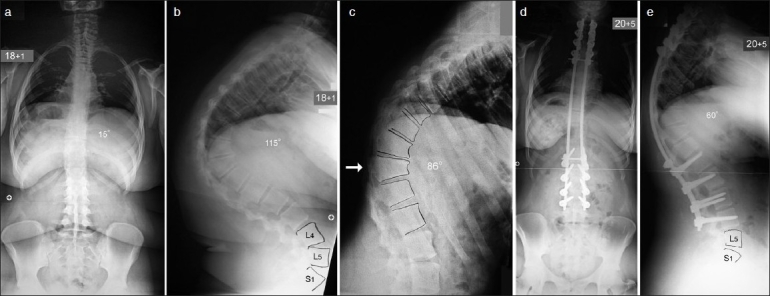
Preoperative posteroanterior radiograph (a) of Patient 2 shows a mild left thoracolumbar scoliosis of 15°. Lateral radiograph (b) shows a severe thoracolumbar kyphosis of 115°. Preoperative supine lateral view in hyperextension against the bolster (white arrow, (c) shows the kyphosis correct to 86o. Posteroanterior (d) and lateral (e) radiographs at 2.3-year-follow-up show no scoliosis and a residual kyphosis of 60°
